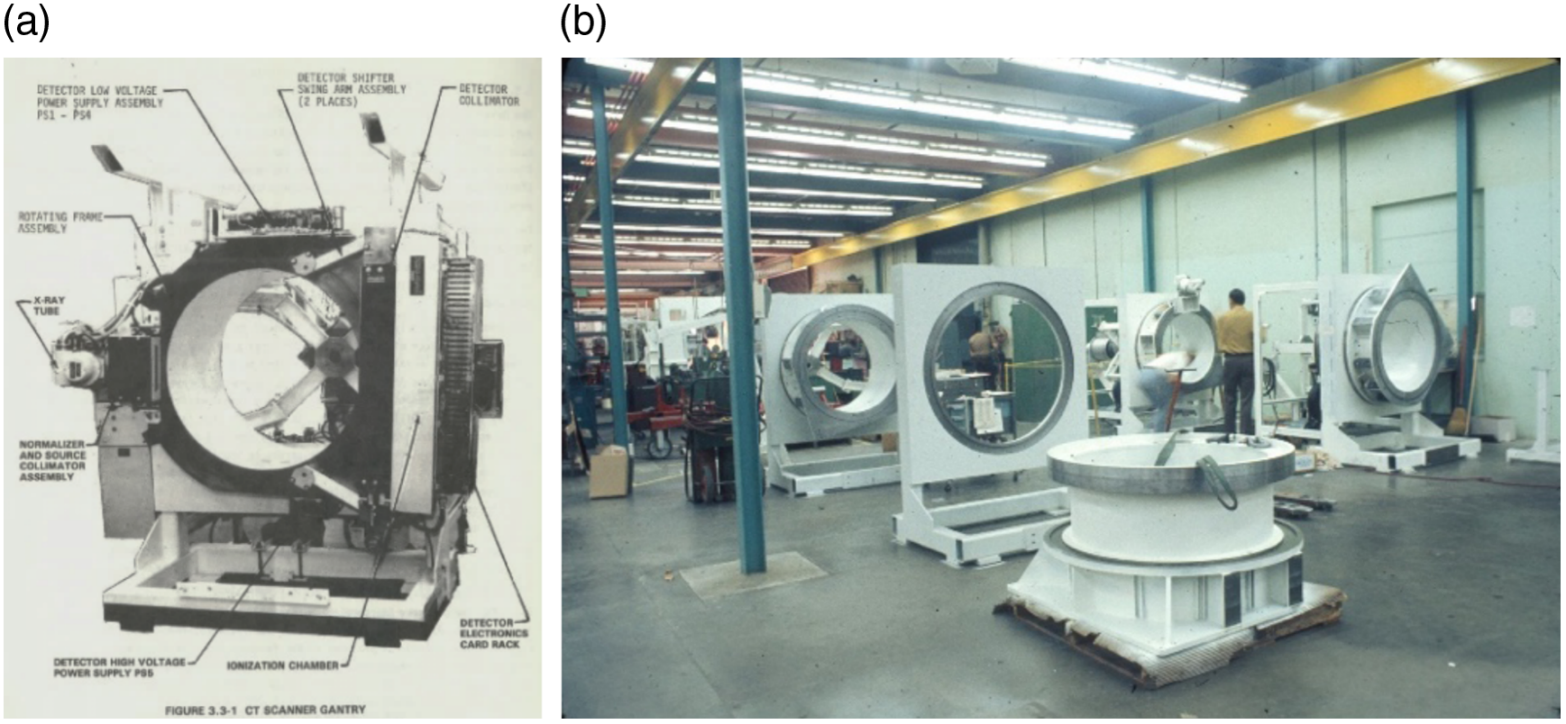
(a) Varian V-360-3 CT scanner with small high voltage slip allowing for continuous rotation, resulting in one side closed off. The high voltage power supply is below the rotating gantry. (b) Manufacturing floor at Varian Instruments. Images courtesy of Ed Shapiro.
